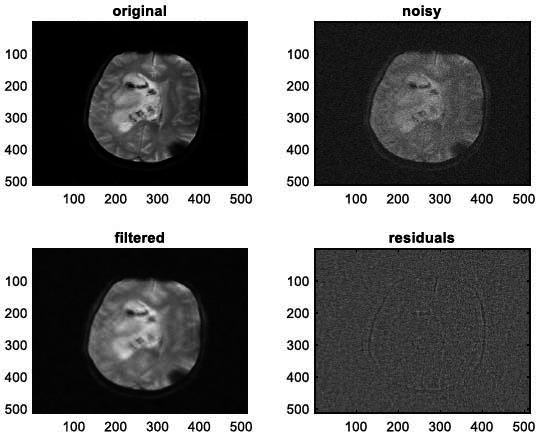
The third example of MRI denoising by applying the proposed image denoising technique.
